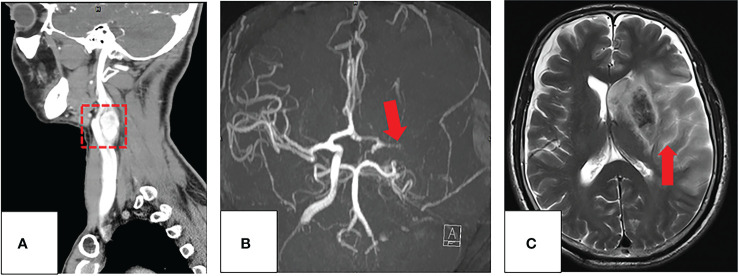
(A–C) Imaging evaluation of a 60-year-old patient with a CBT and occlusion of the left ICA post-surgery. (A) The coronal image of the preprocedural CT angiography of CBT (red box). (B) After surgical resection with preoperative embolization of CBT (red arrow), the patient had a sudden right limb weakness. The axial maximum-intensity-projection images from an MRA demonstrate occlusion of the left middle cerebral artery. (C) T_2_WI demonstrates a large acute infarction involving almost all the left middle cerebral artery territory (red arrow).
